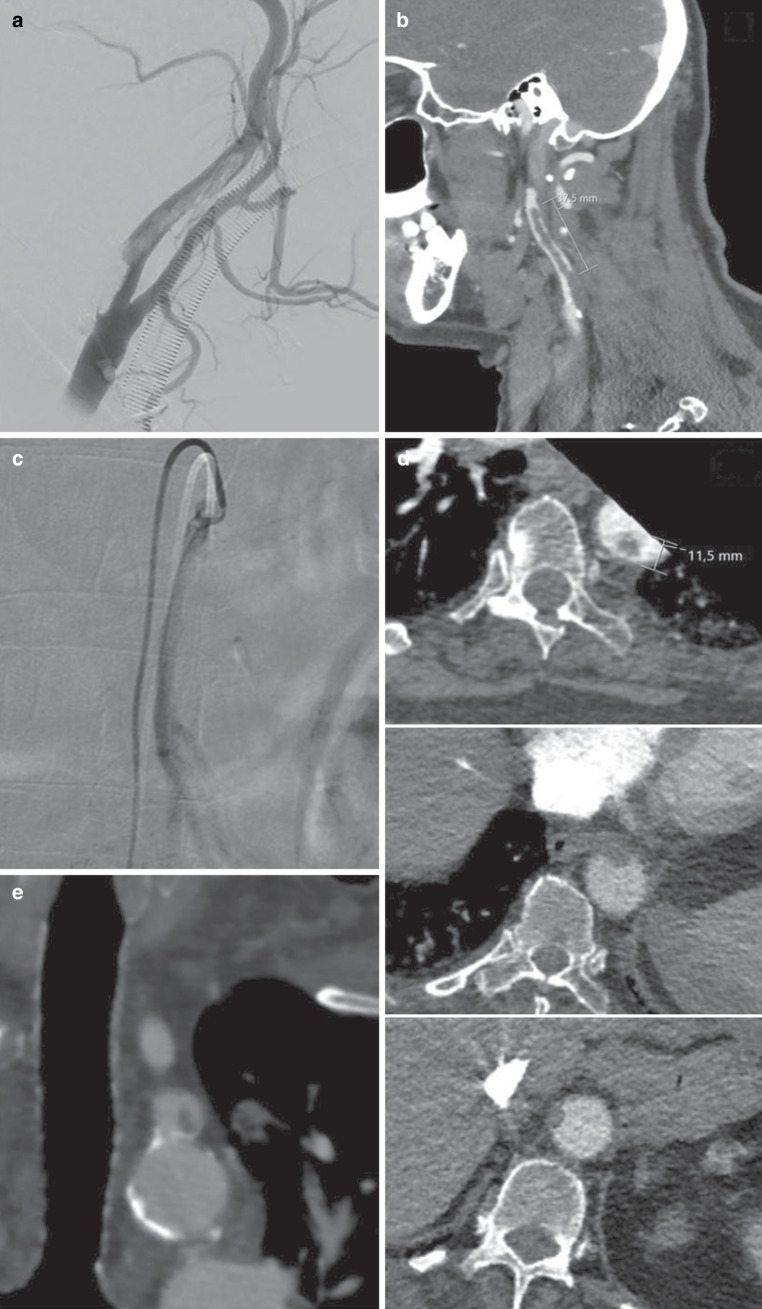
Imaging morphology of ATE co-occurring with COVID-19 infection. a Digital subtraction angiography (DSA) shows a massive thrombus of the internal carotid artery, that is partially wall-adherent to a non-ulcerating plaque and with a long free-floating portion inside the internal carotid artery. b CT-angiography (CTA) of the internal carotid artery in the same patient shows the ≈ 3.7 cm long floating portion of the thrombus. c DSA shows another example of a partially wall-adherent and floating thrombus without visible atherosclerotic changes of the superior mesenteric artery. d CTA of the aorta shows multiple wall-adherent thrombi. e CTA shows partially wall-adherent and floating thrombi of the left subclavian artery
